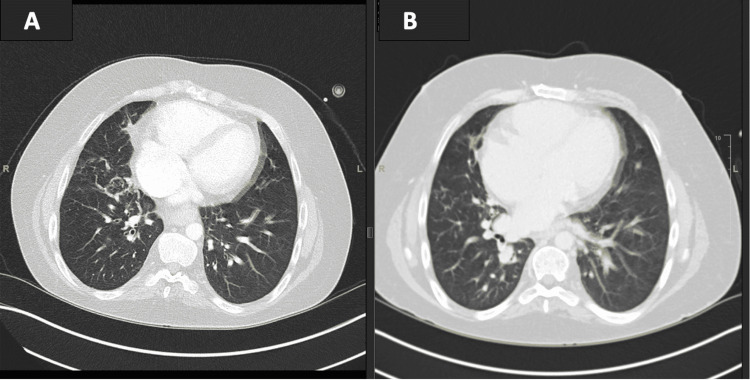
CT Chest with Comparison Case imaging shows bronchiectasis and scarring of the lung bases from prior pulmonary infections during childhood and young adulthood. Figure 1A is from May 2020. Figure 1B is from July 2021.
